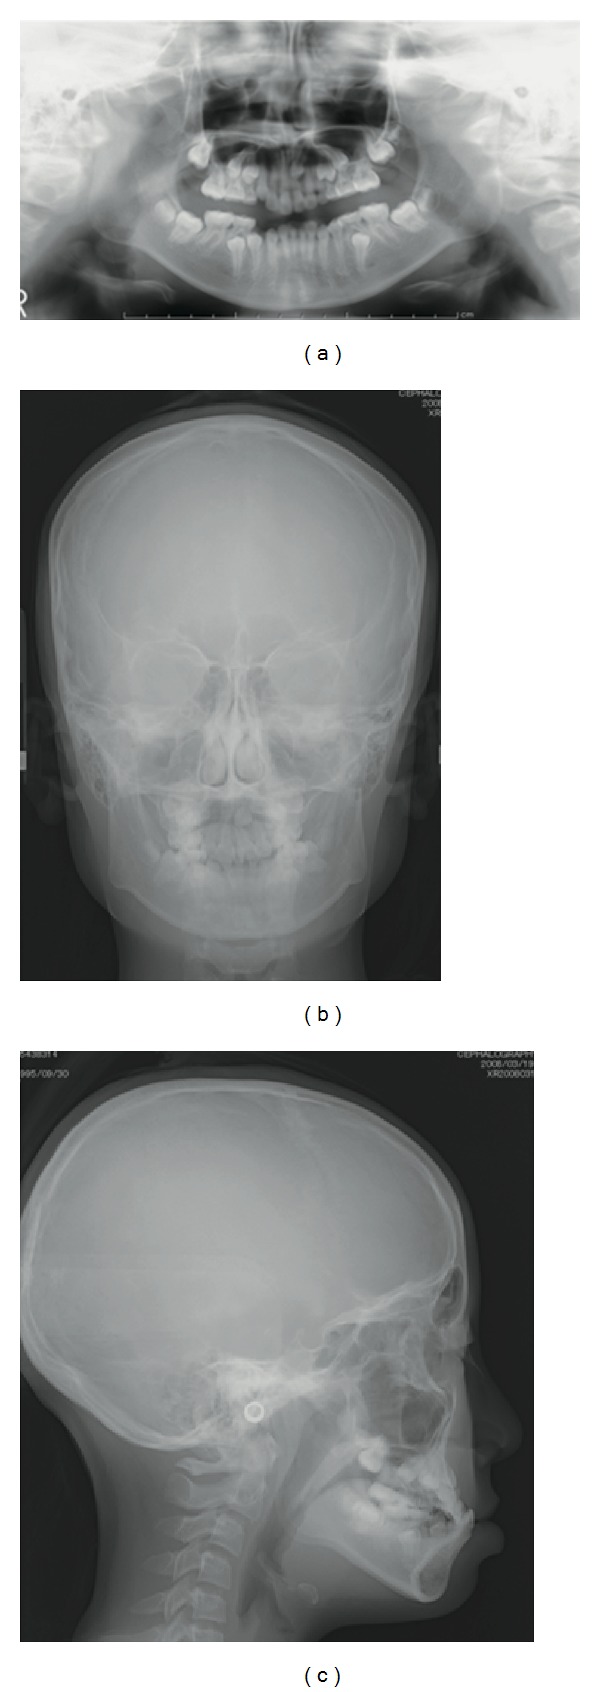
Radiographic appearance at the age of 12 years and 6 months: (a) panoramic radiograph, (b) frontal cephalometric radiograph, and (c) lateral cephalometric radiograph.
